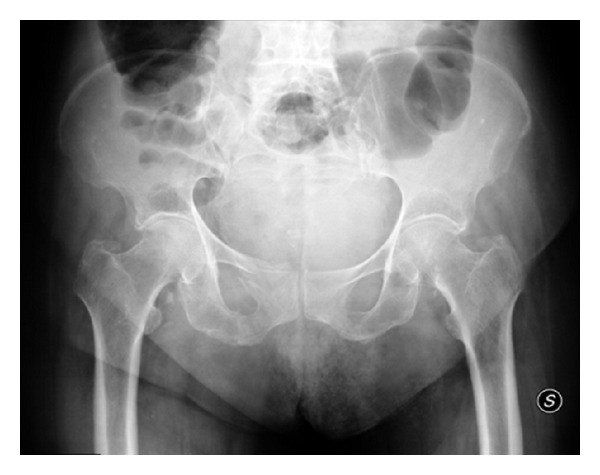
Anteroposterior pelvic radiograph revealing right superior and inferior pubic rami fractures.
